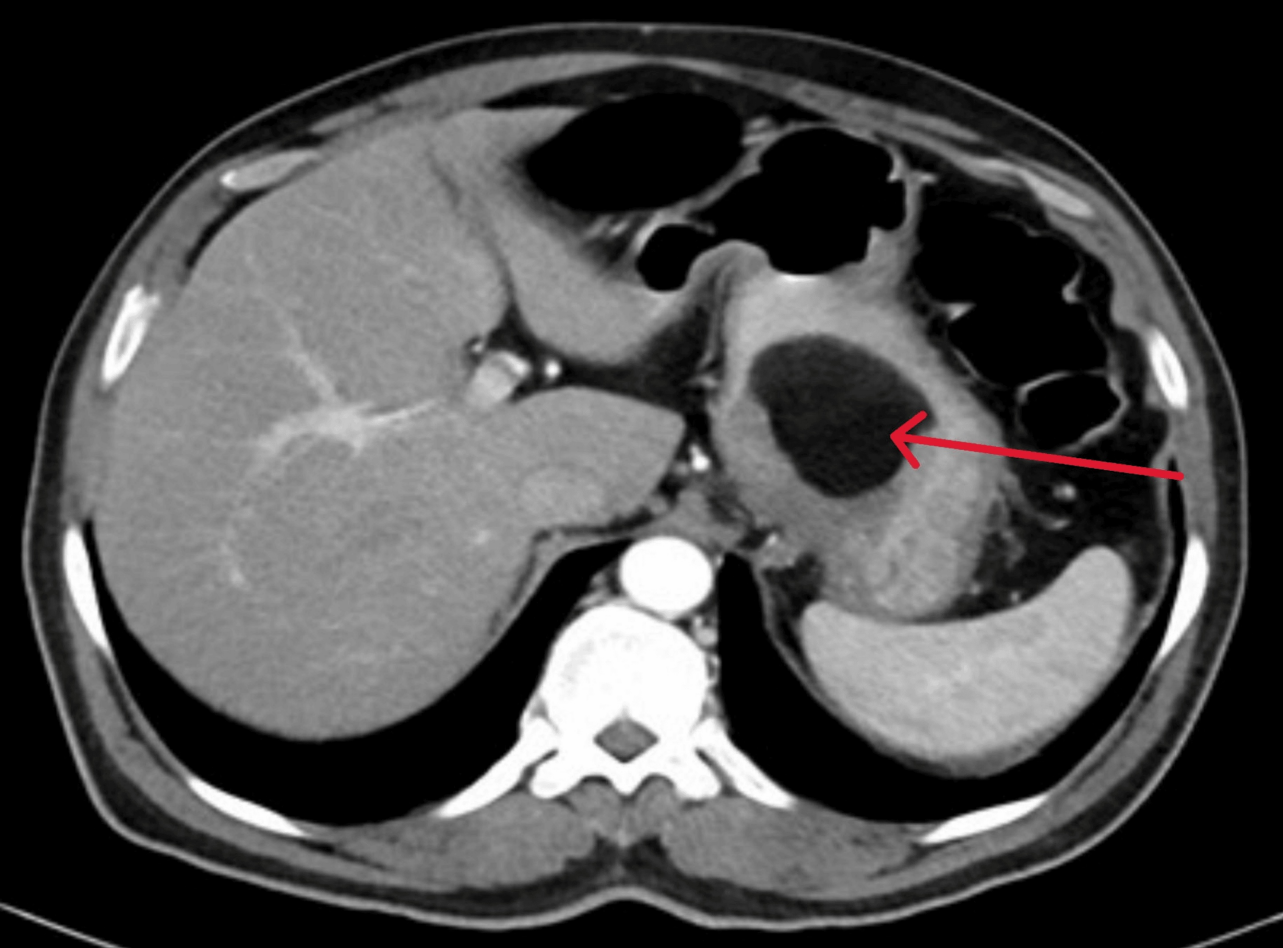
CT scan-axial view, fat-containing intramural subepithelial mass lesion CT scan (axial view) showing a large mass lesion in the fundus and cardia of the stomach, measuring 6.5 x 6.2 x 5.4 cm (red arrow)
